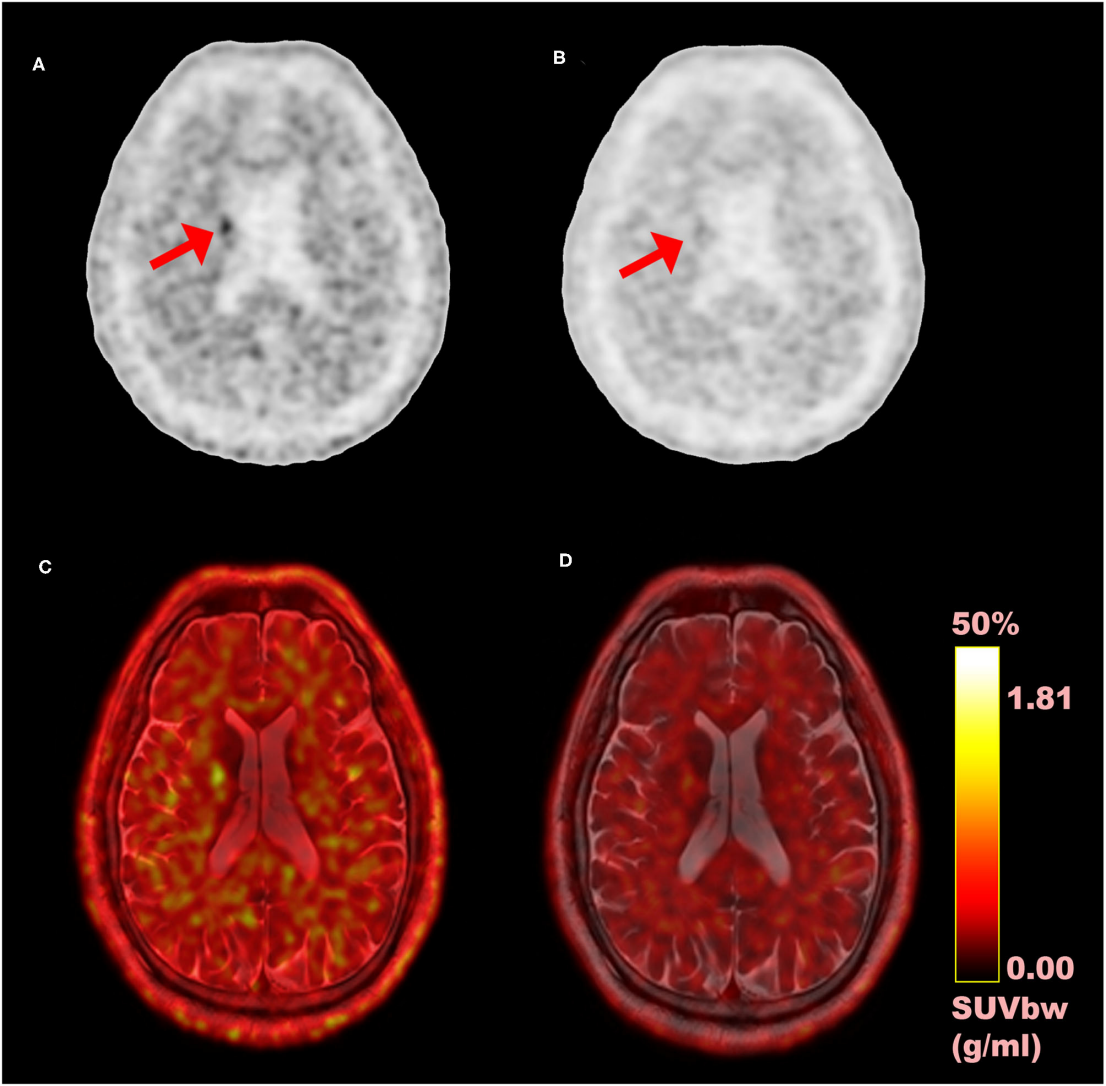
Comparison of time-of-flight (TOF) vs. non-TOF ^11^C-PiB PET images; (A) TOF reconstructed PET image (axial plane); (B) non-TOF reconstructed PET image (axial plane); (C) fusion image of TOF PET and MRI; (D) fusion image of non-TOF PET and MRI; red arrows: shows the signal enhancement difference in TOF and non-TOF PET images in ^11^C-PiB PET images.
